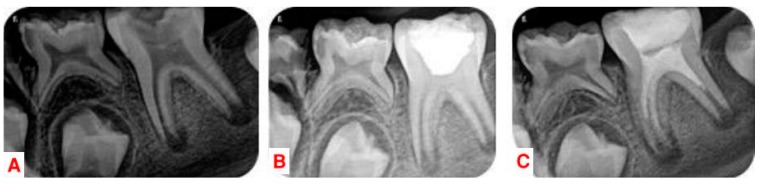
(A) Preoperative radiograph. (B) Placement of Ca(OH)_2_ in the pulp chamber. (C) Postoperative radiograph after root canal disinfection, bleeding induction, placement of MM-MTA over blood clot, placement of coronal restoration.
